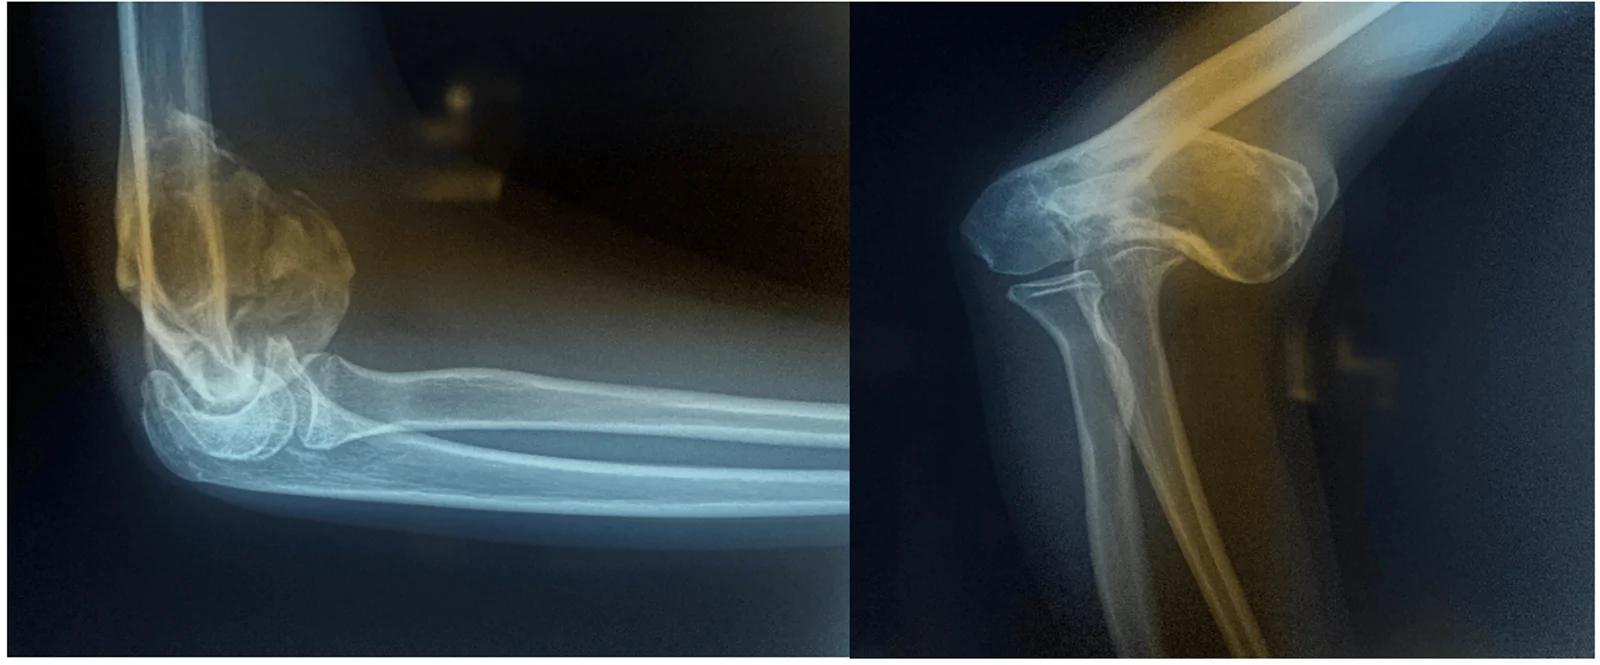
Anteroposterior and lateral plain radiographs of the right elbow after six months of denosumab therapy, showing marked regression of the osteolytic lesion at the medial epicondyle of the distal humerus, with progressive remineralization of the tumor matrix, consolidation, and formation of a peripheral neo-cortical shell, consistent with a favorable radiological response to anti-RANKL therapy RANKL, receptor activator of nuclear factor-κB ligand
